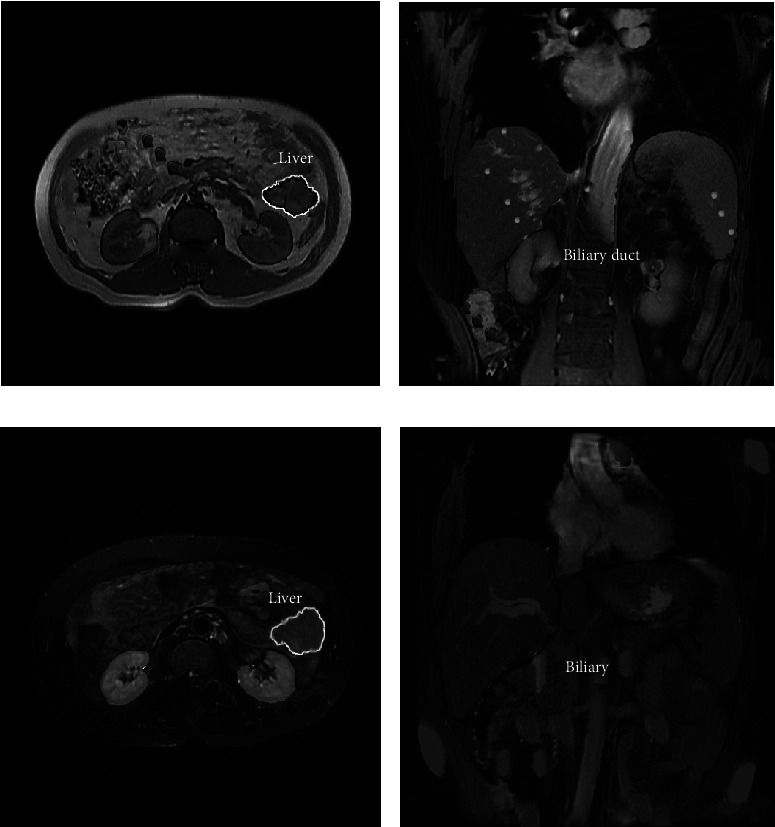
MRI manifestations of ICP pregnant women. (a) Patient 1, female, 35 years old, bile duct dilation with cholestasis in transverse position; (b) patient 1, female, 35 years old, with dilated bile ducts and coronal position with cholestasis; (c) patient 2, female, 29 years old, undilated bile duct with cholestasis in transverse position; (d) patient 2, female, 29 years old, coronal position with undilated bile duct and cholestasis.
